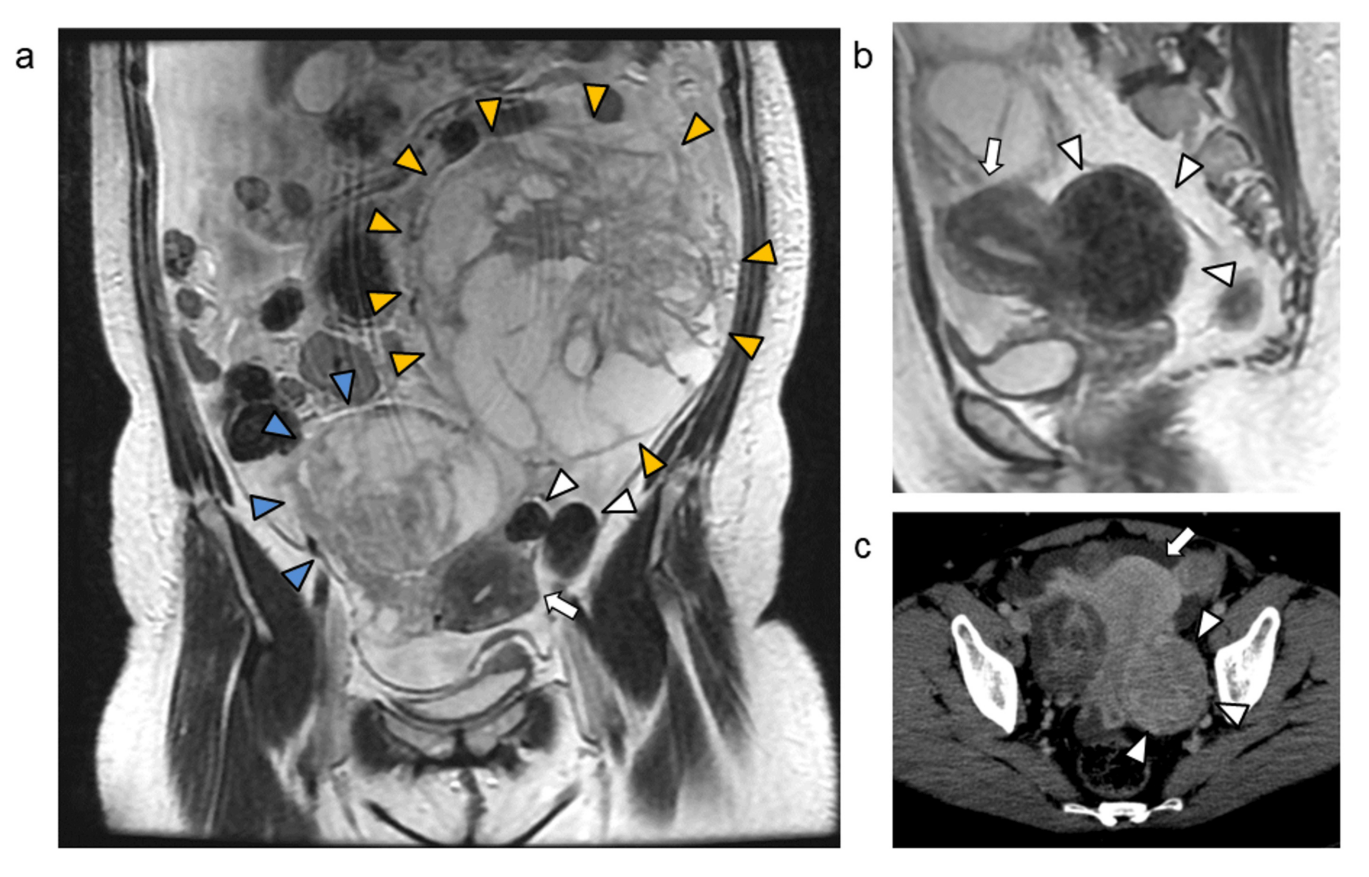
MRI and CT findings at initial diagnosis (a) Abdominal contrast-enhanced coronal T2-weighted MRI showing large masses with multilocular cystic components and mixed solid areas in both adnexa (blue arrowheads, right adnexa; orange arrowheads, left adnexa). Two subserosal leiomyomas (2 and 5 cm) (white arrowheads) are visible on the left side of the uterine fundus (white arrow, uterus). A 7-cm subserosal leiomyoma extending from the lower part of the uterine body to the cervix is depicted on sagittal T2-weighted MRI (b) and axial contrast-enhanced CT (c) (white arrow: uterus).
